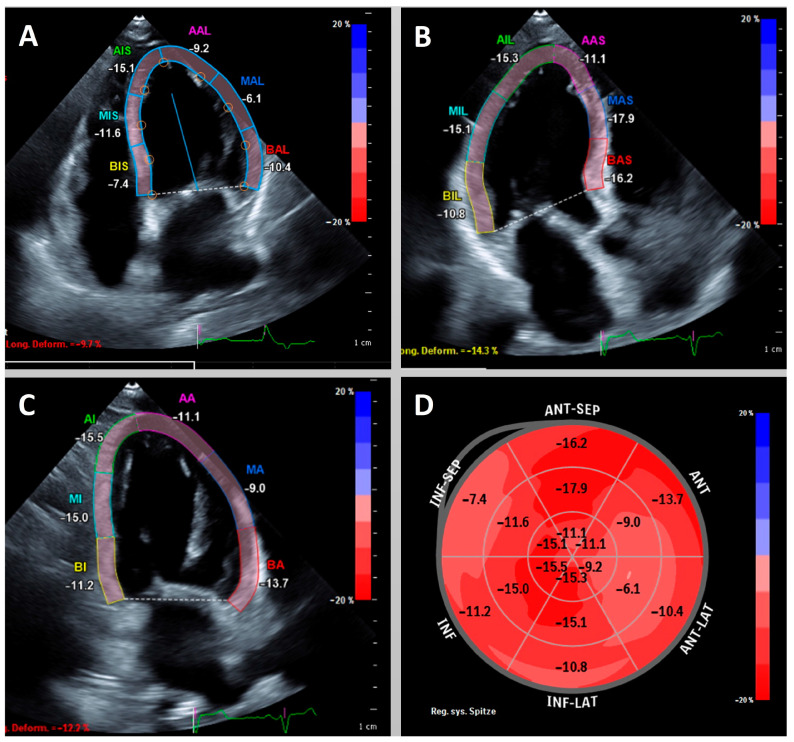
Example of a patient with reduced left ventricular global longitudinal strain (GLS) of −12.1%. Apical 4-, 3- and 2-chamber view (A–C) with highlighted left ventricular myocardium. Bullseye plot (D) shows globally reduced longitudinal strain with accentuation basally septal and midventricular anterior/anterolateral.
